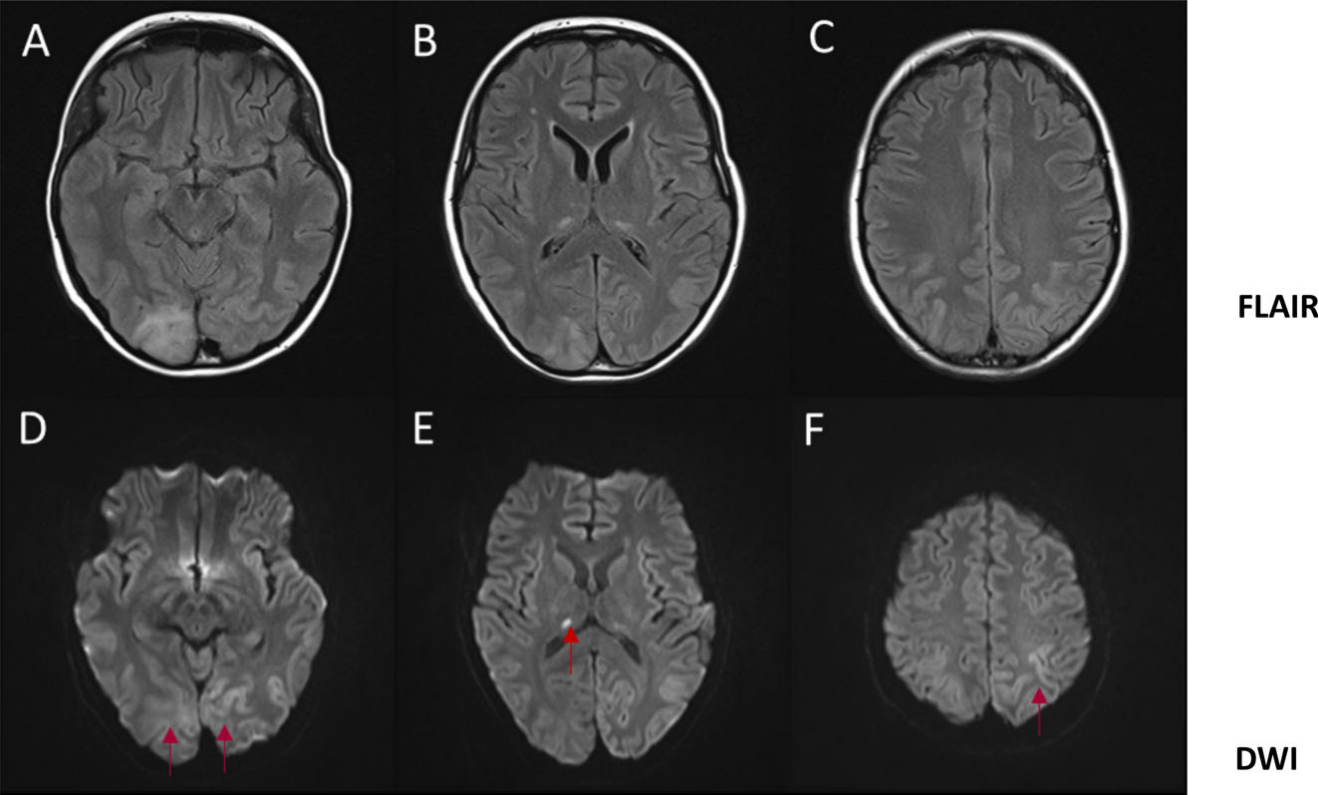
Axial view of MRI head. FLAIR‐sequence (A–C) shows hyperintense lesions involving the right occipital lobe, right thalamus, and bilateral parietal lobes. DWI sequence (D–F) shows restricted diffusion in the bilateral occipital lobes, right thalamus, and left parietal lobe (red arrows) with increased ADC map (not shown), suggestive of vasogenic edema.
